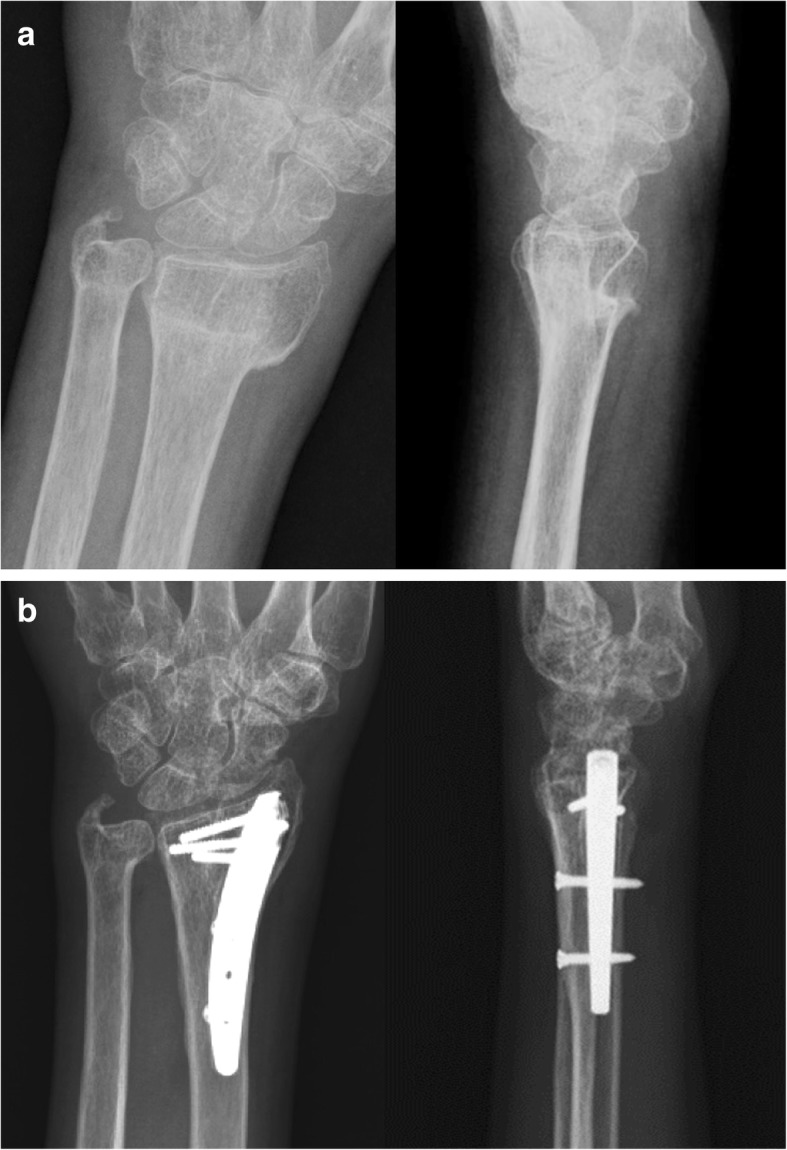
A 63-year-old female patient with distal radius fracture of 16 weeks. a Preoperative radiographs showing malunion, posteroanterior, and lateral projections. b Postoperative radiographs with intramedullary nail 2 years after surgery follow-up showing posteroanterior and lateral projections
